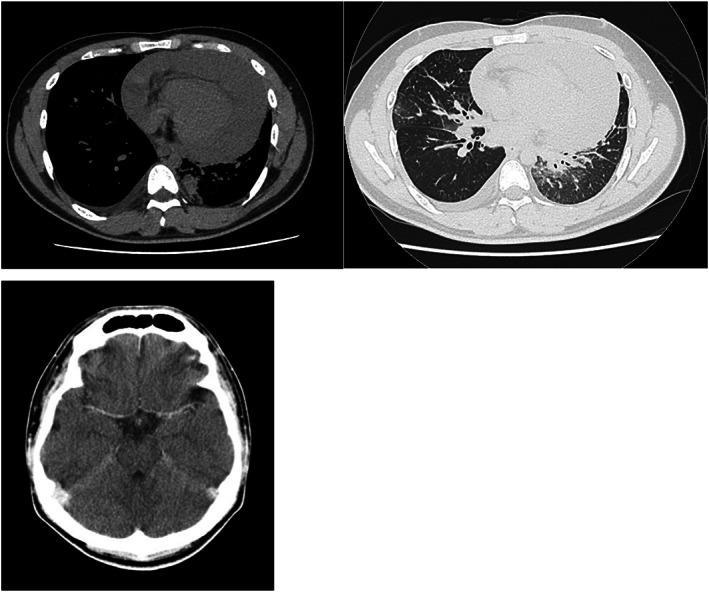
Initial computed tomography (CT) scan showing pericardial effusion and multiple granular shadows and consolidations in both lungs with no evidence of central nervous system metastasis.
